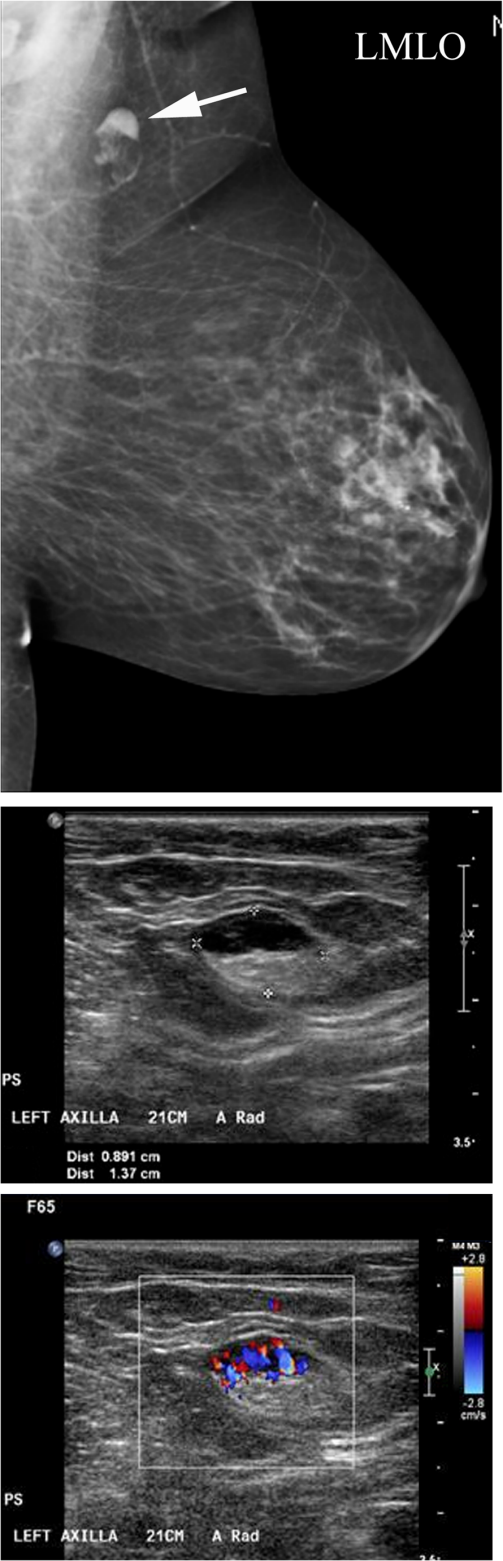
A 54-year-old female with remote history of right breast cancer and a left knee sarcoma now for screening. a MLO view demonstrates a focally thickened non-enlarged left axillary lymph node. b Ultrasound confirms a 1.4-cm lymph node with focal cortical thickening of 6 mm and (c) internal vascular flow. FNA was performed for diagnosis. Aspirates were sent for routine cytology and flow cytometry. Final diagnosis: reactive lymph node
